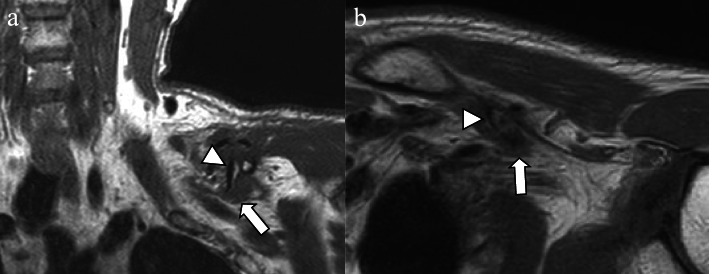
Magnetic resonance imaging (a) T1-weighted coronal; (b) axial brachial plexus magnetic resonance imaging: brachial plexus (arrow) was compressed by the displaced comminuted fracture fragment (arrowheads)
